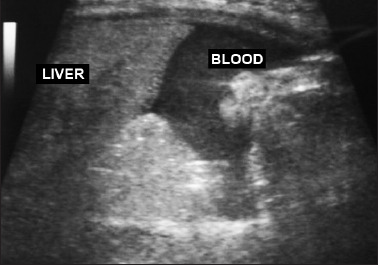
Abdominal ultrasonography showing hemoperitoneum: blood accumulation can be seen near the liver.
